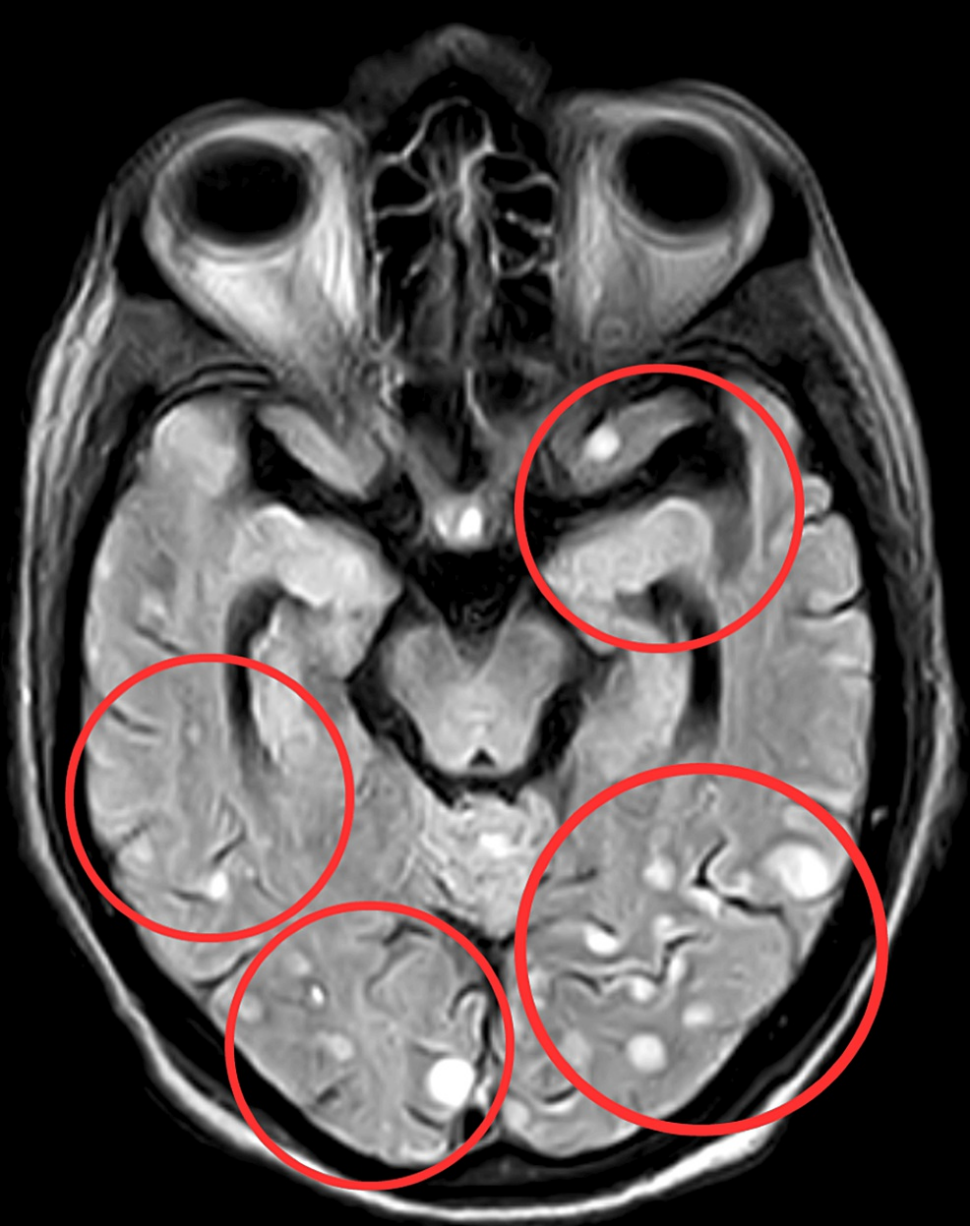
MRI of the brain The red circles show variable-sized round to oval lesions noted in the bilateral cerebral hemisphere and bilateral cerebellar hemisphere MRI: magnetic resonance imaging
